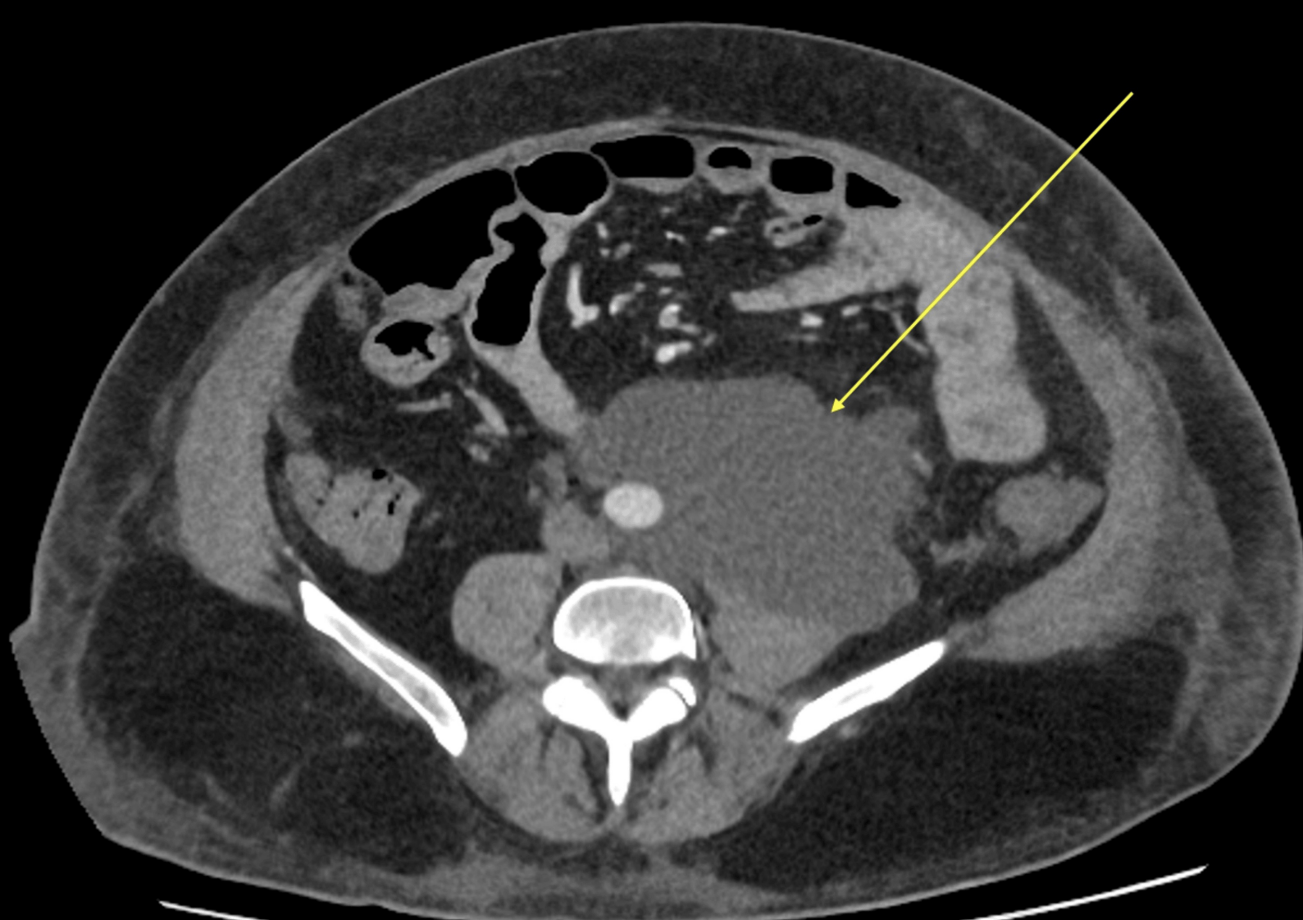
Axial CT image of the abdomen and pelvis demonstrating a large, low-attenuation fluid collection along the abdominal aorta, with no evidence of leak. The collection abuts the left psoas muscle and lies close to the left ureter, with no current ureteric obstruction.
